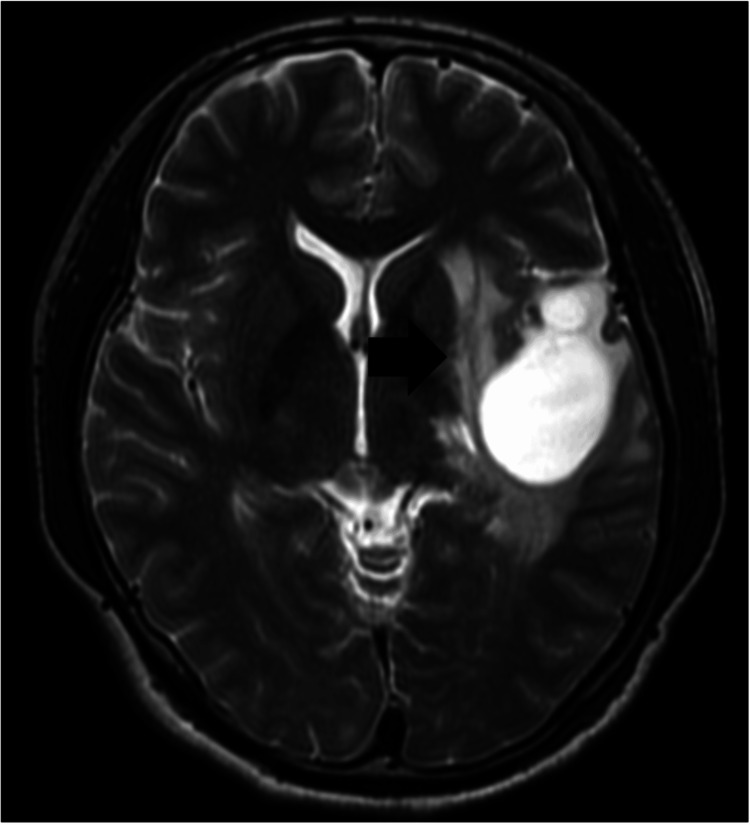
Axial magnetic resonance image of T2-weighted image showing perifocal edema (black arrow) getting bigger than 22 months ago, when the cyst was diagnosed.
